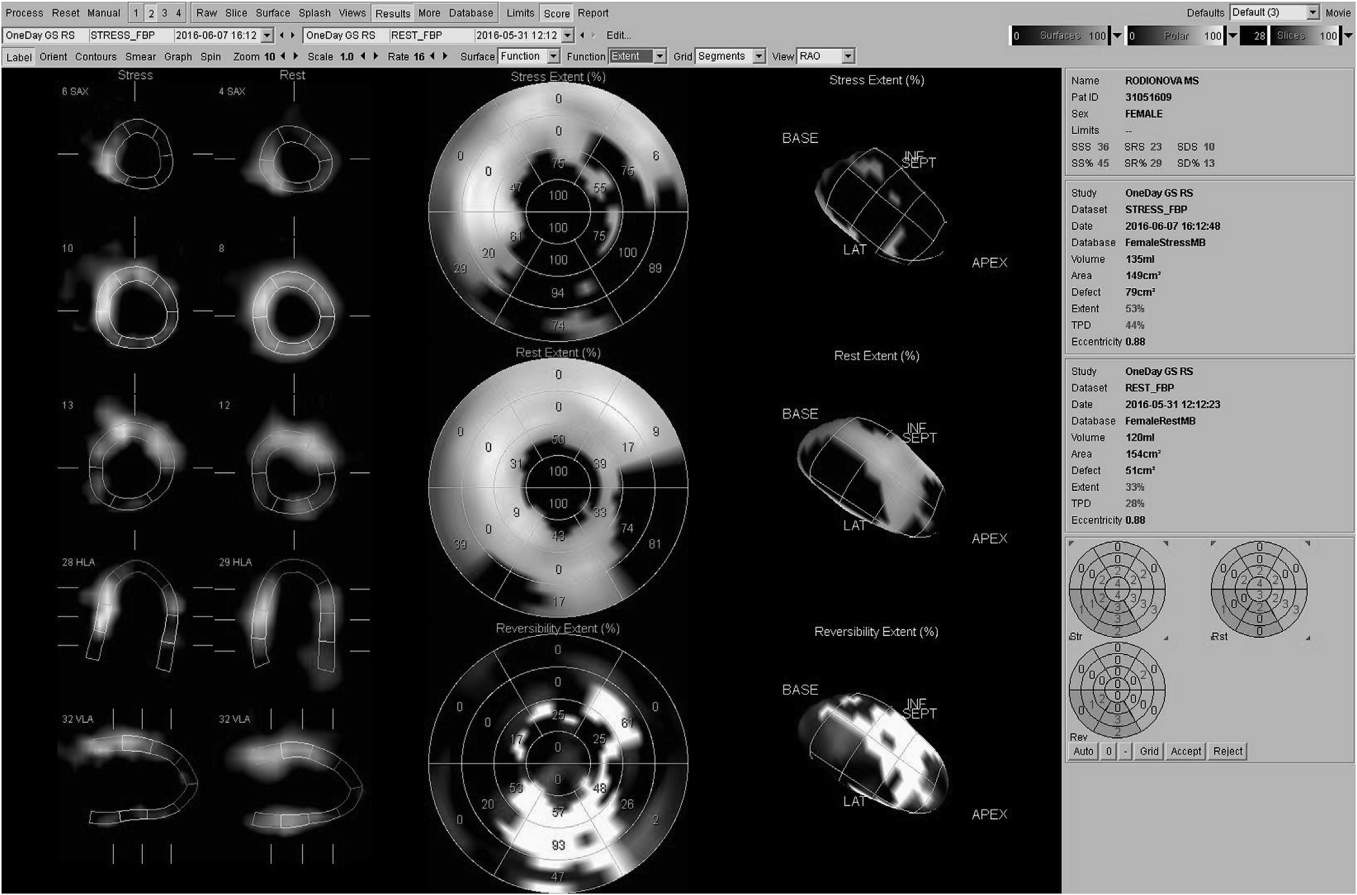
Radionuclide myocardial perfusion scintigraphy results.
